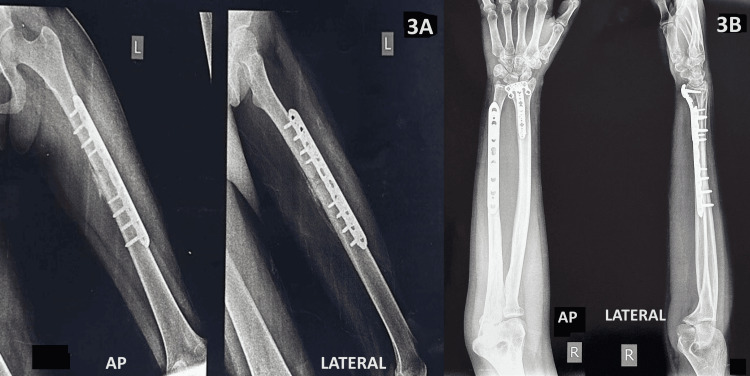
Postoperative plain radiograph. (A) Open reduction internal fixation with plating for femur shaft fracture in anteroposterior (AP) and lateral views. (B) Open reduction internal fixation with plating for ulna shaft fracture and distal end radius fracture in AP and lateral views.
